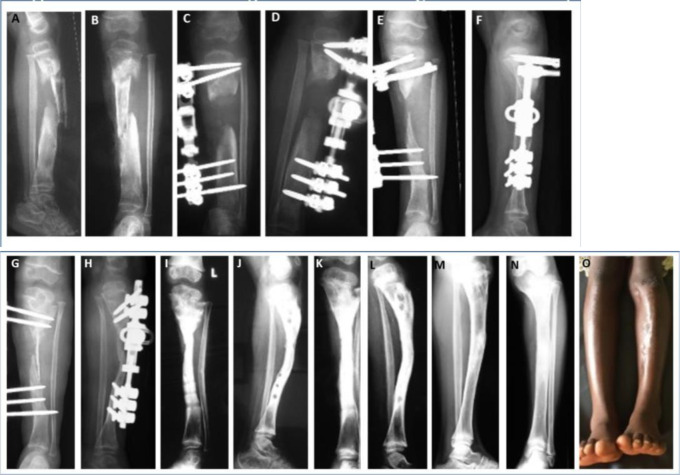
A 2-year-old girl presented 2 months after spontaneous onset of infection, with an exposed sequestrum, pathological fracture and loss of soft tissues (a, b). Sequestrectomy and external fixation were carried out (c, d), leaving the skin to heal by secondary intention. Pin loosening required a change in the frame, but two proximal screws were erroneously inserted into the upper physis (e, f). Six months after the initial debridement, a tibial cortex strut was inserted and the fixator was replaced (g, h). Radiographs are shown at 5 (i, j) and 7 (k, l) months of grafting. Sound graft incorporation and bone modelling may be observed. Seven years later, at the age of 12 years (m, n, o). Closure of the upper tibial physis led to a LLD of 2 cm and deformity of the tibial plateau. However, good bone and functional results were achieved in the long term.
